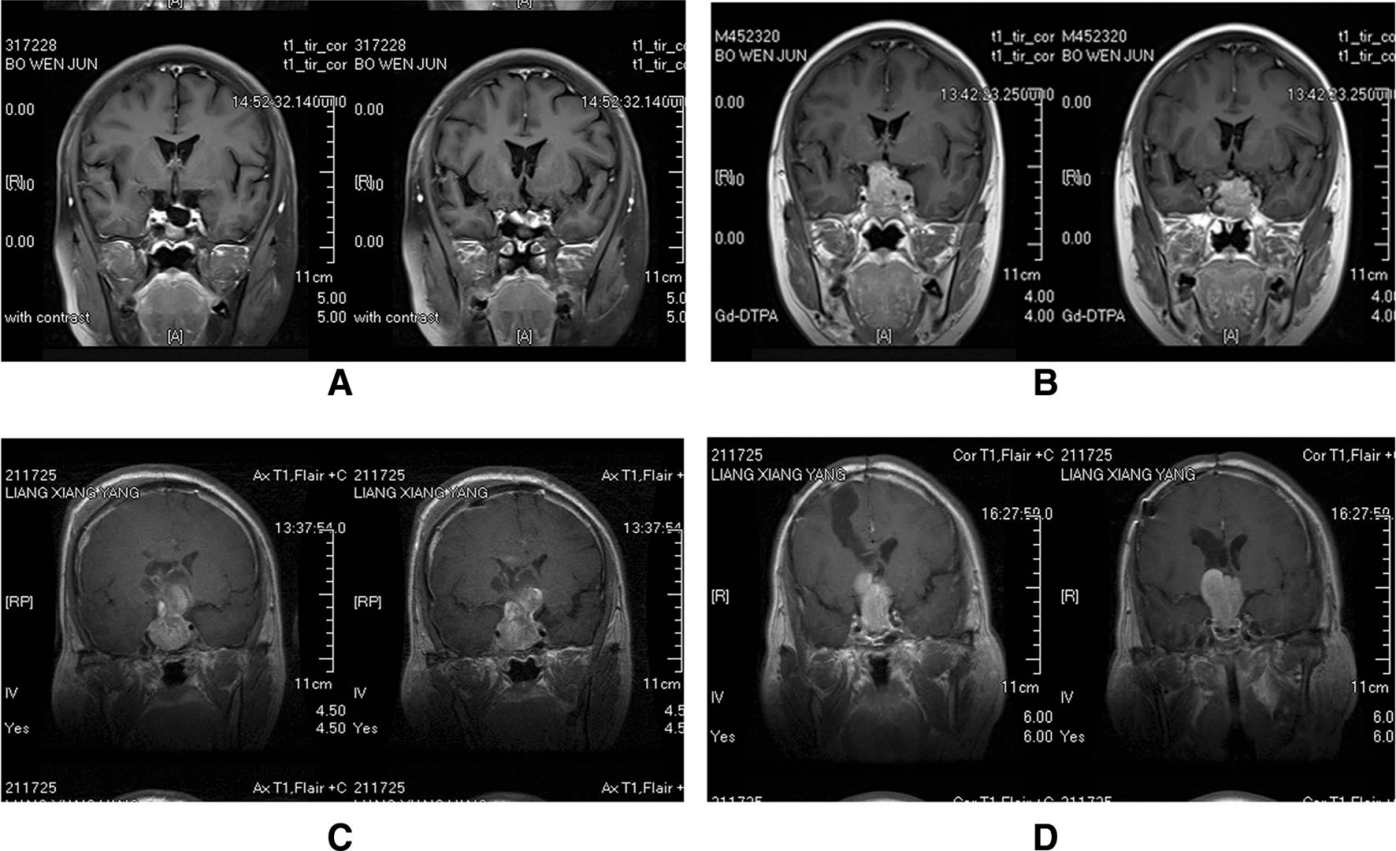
a First postoperative MR image demonstrating complete tumor removal in a 30-year-old women. b MRI scan obtained 24 months after surgery shows a distinct tumor recurrence in the sellar. c First postoperative MR image demonstrating incomplete tumor removal in a 41-year-old men. d MRI scan obtained 58 months after surgery shows a distinct tumor regrowth in the sella
